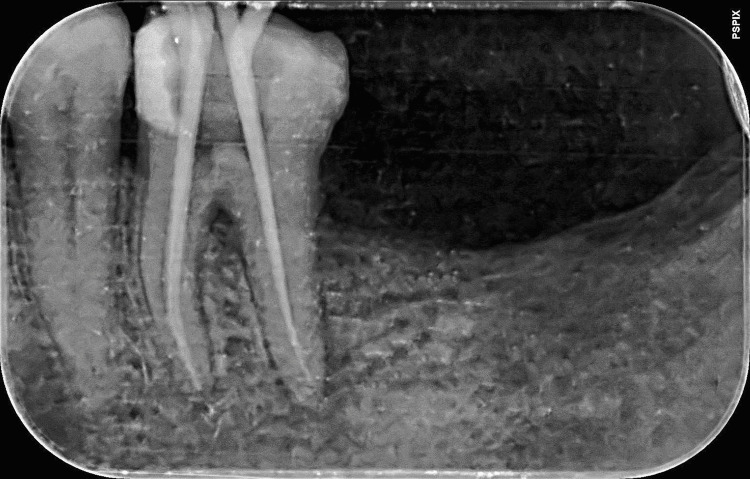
Master cone fit check A radiograph demonstrating a master cone fit check for the canal obturation procedure.
